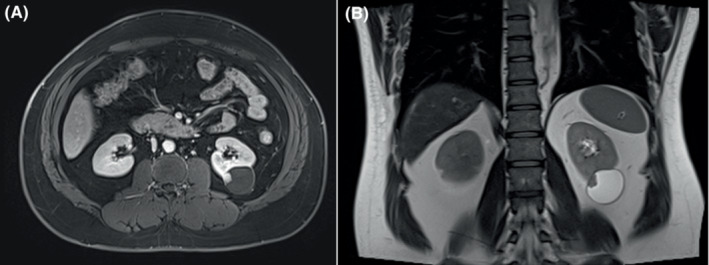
Magnetic resonance imaging of succinate dehydrogenase‐deficient renal cell carcinoma. (A) T1‐weighted imaging showed that the cystic‐solid mass had short signals and the solid component of the mass had long signals. (B) T2‐weighted imaging showed that the mass had long signals and the solid component of the mass had short signals.
